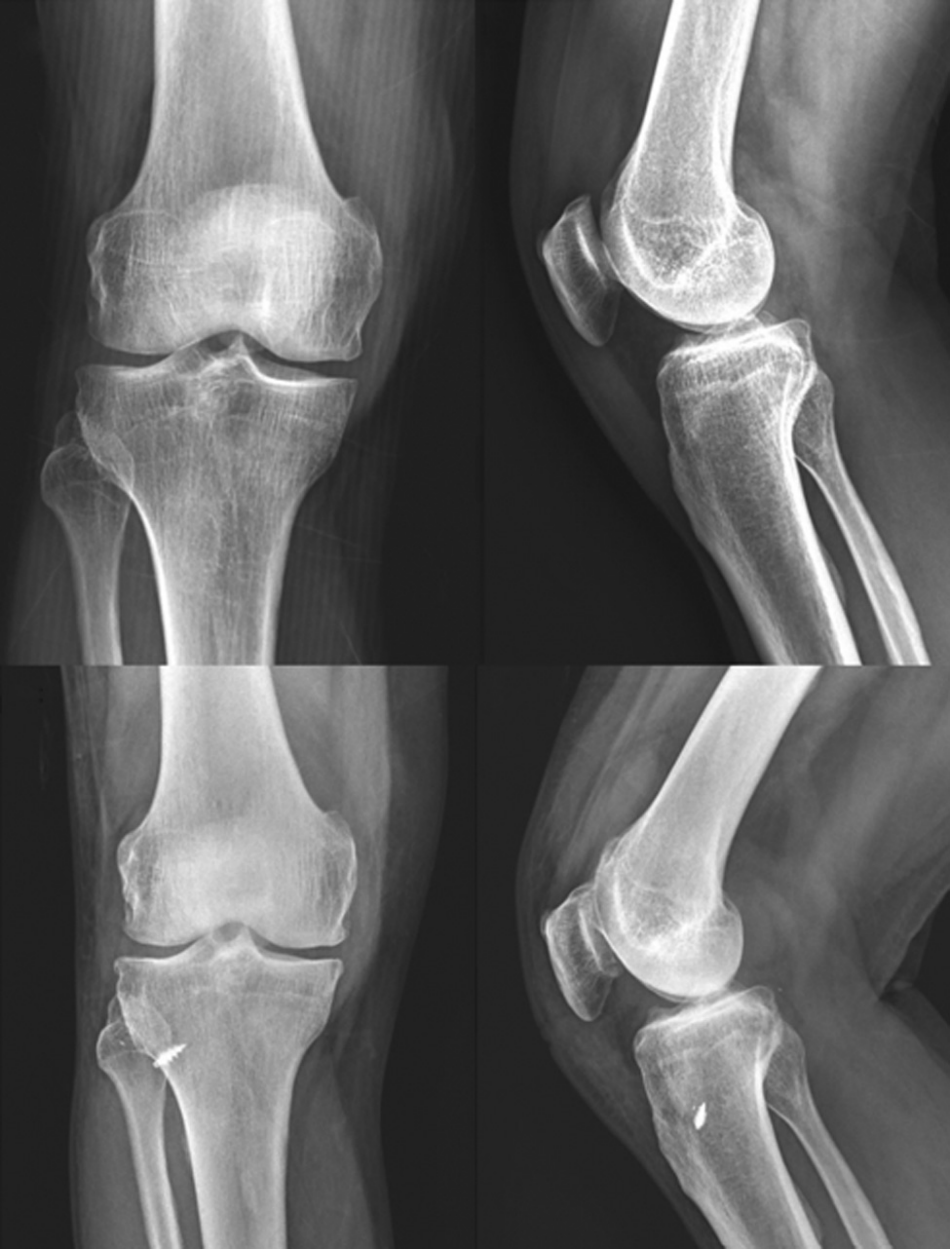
Preoperative and postoperative follow-up radiographs.
